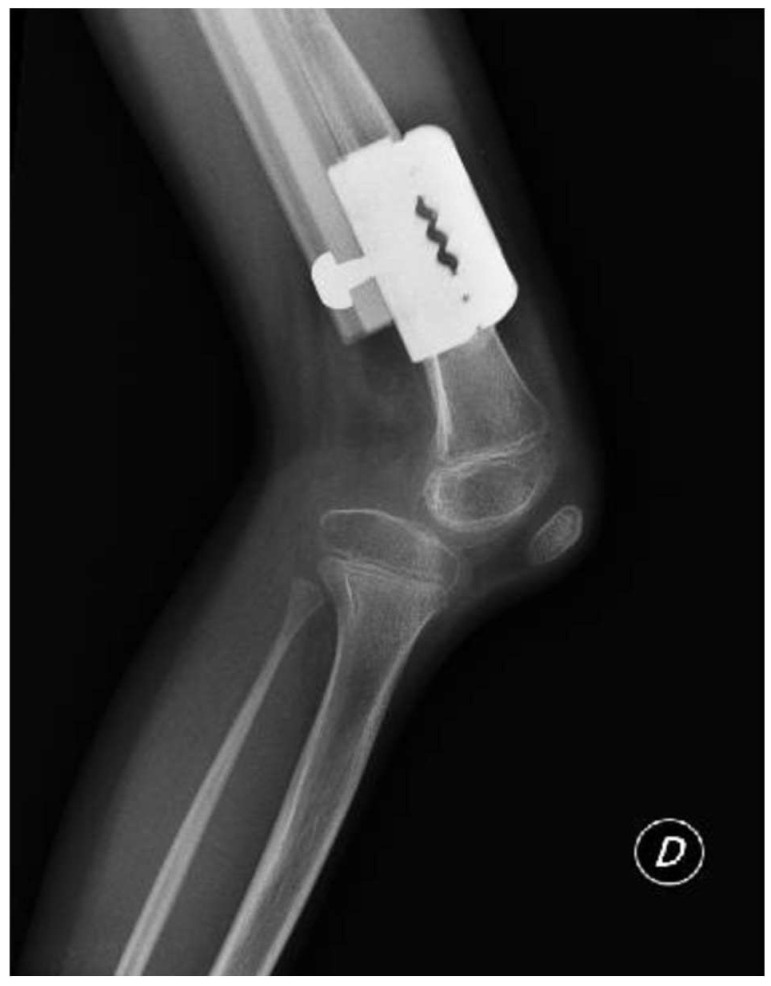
Lateral projection radiographs of the right knee showing posterior subluxation of the tibia with respect to the femoral condyles.
